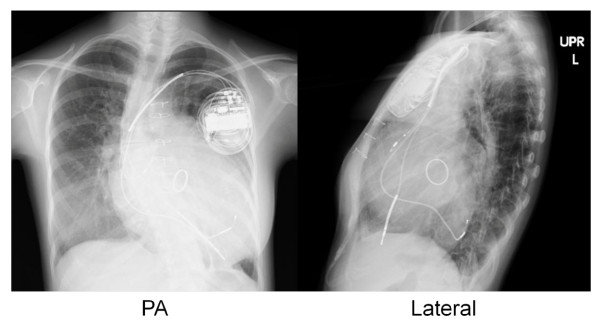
The chest X-ray (PA and Lateral projection) after the CRT placement.
